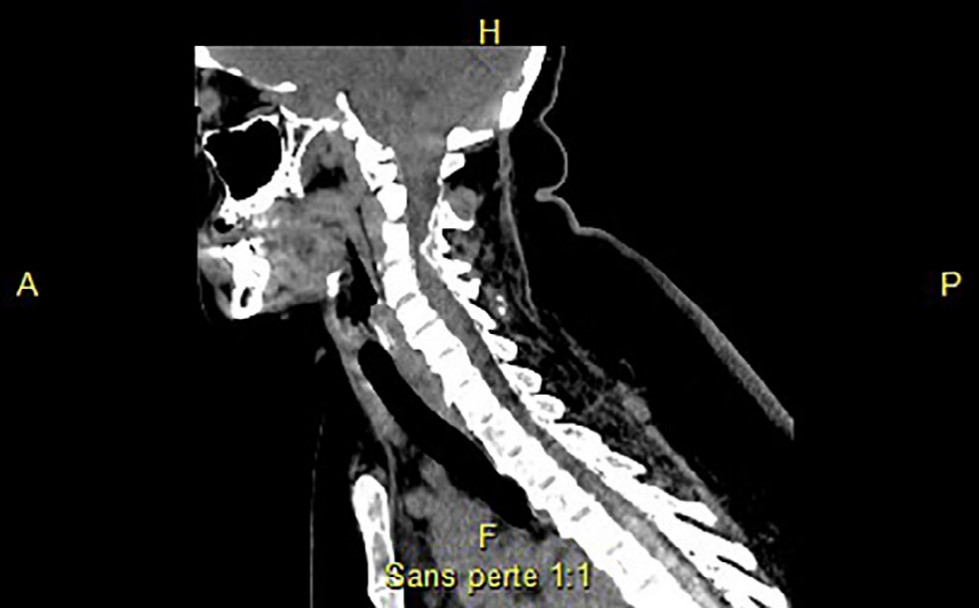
CT scan of the cervical spine, sagittal sections: ossifications of the yellow ligament, more pronounced in C_3_–C_4_.
